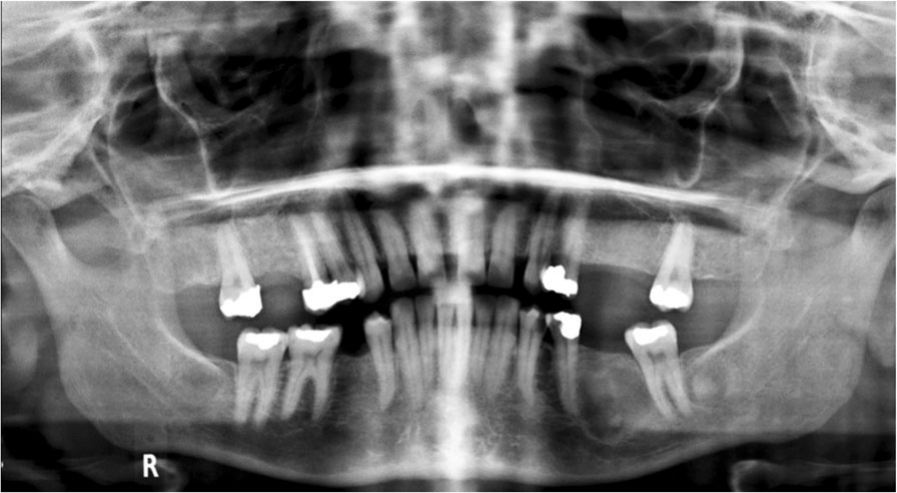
On the orthopantomogram (OPG), there is a single well-defined, corticated, lucent lesion in the body of the left mandible between the premolar and only remaining molar tooth
